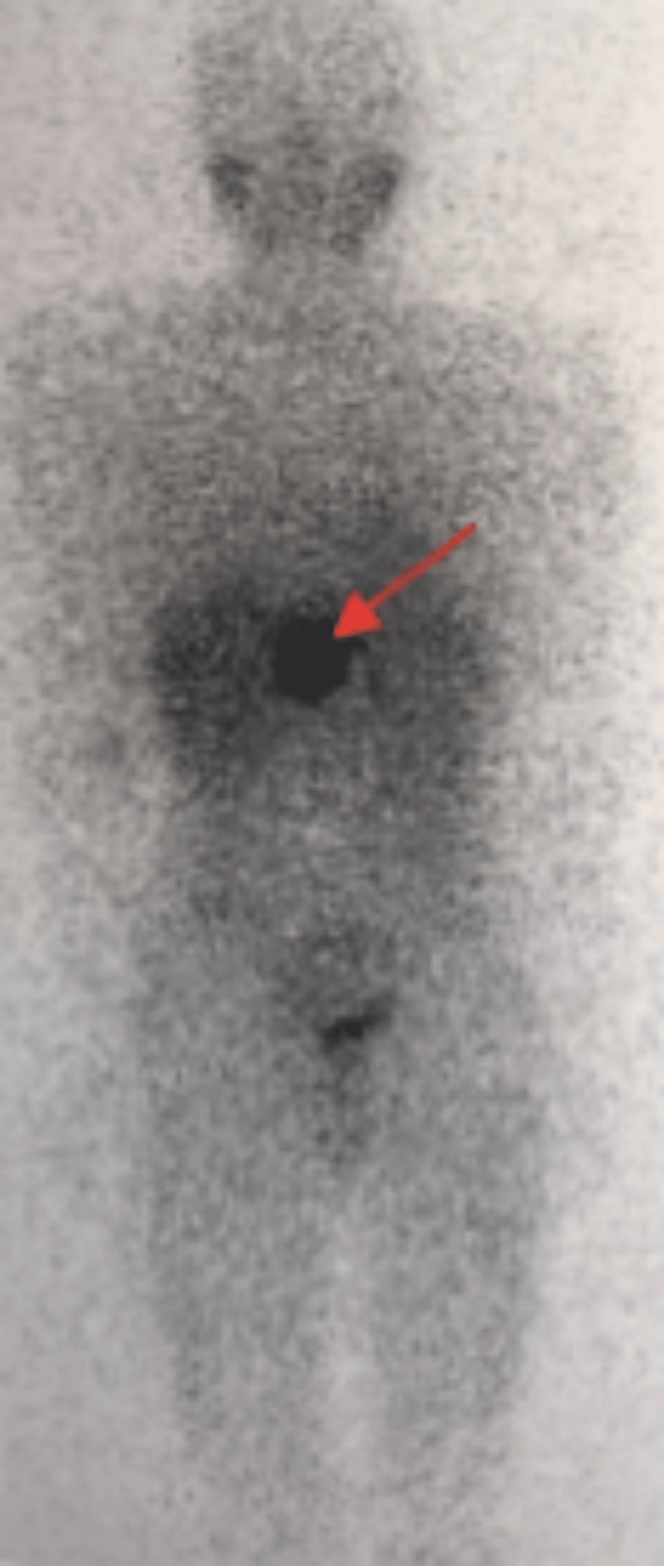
MIBG scintigraphy shows clear fixation corresponding to the retro-hepatic mass described in the MRI MIBG, metaiodobenzylguanidine; MRI, magnetic resonance imaging.
